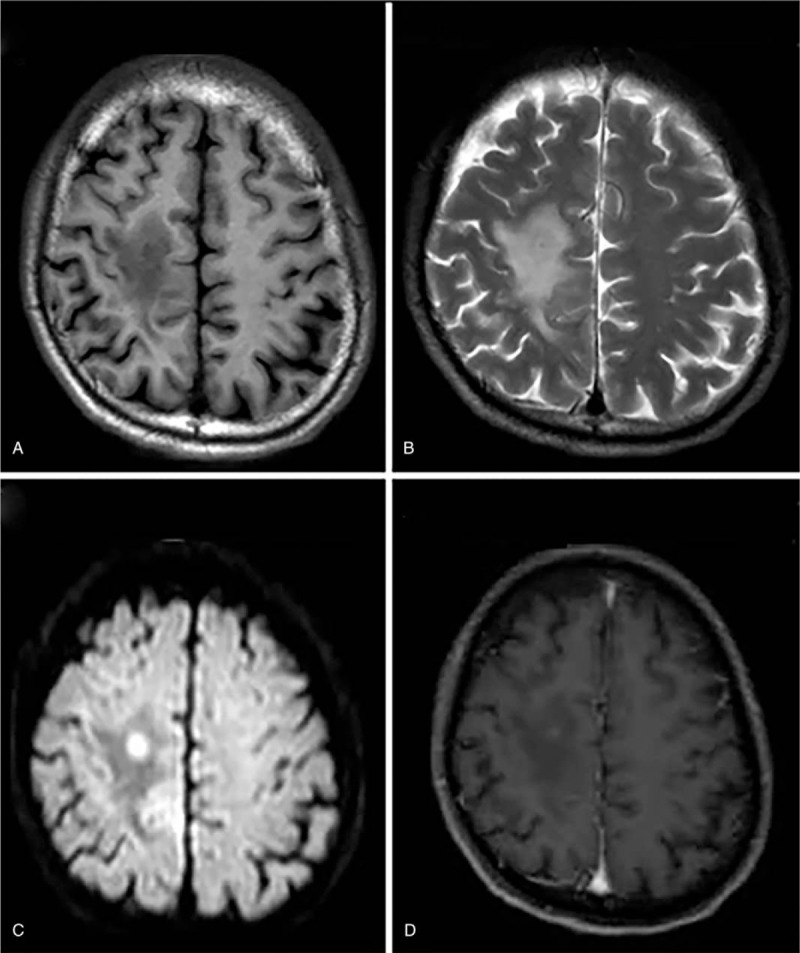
Brain MRI. A, B, T1WI and T2WI showed lesions with peripheral edema involving white matter of right hemisphere. C, D, Diffusion-weighted imaging (DWI) signals were high with mild enhancement. MRI = magnetic resonance imaging.
